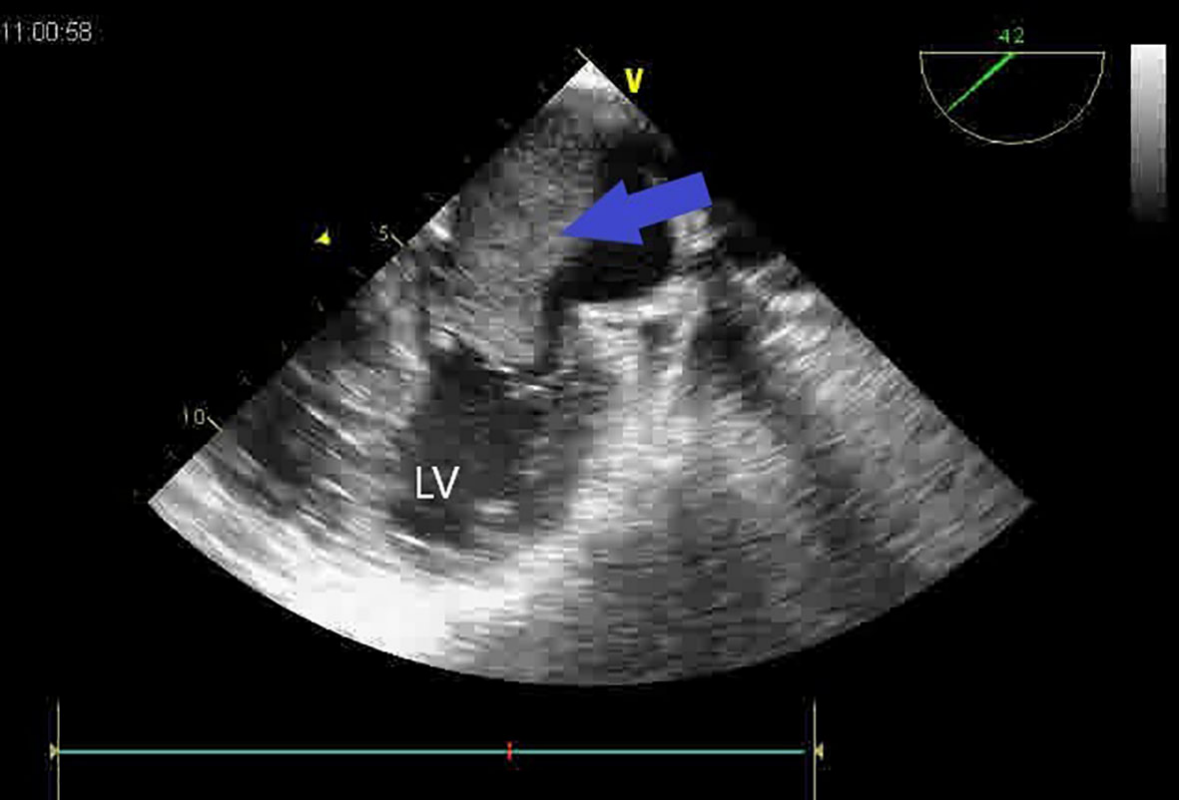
Transesopheagal echocardiography showing a large mass, occupying nearly all the left atrium (blue arrow) and mitral obstruction.
